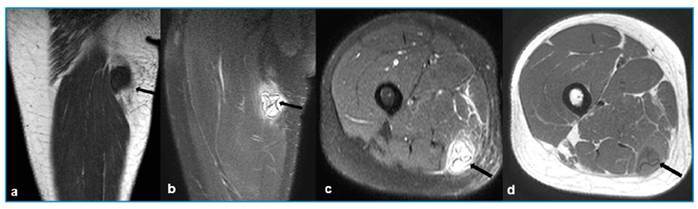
a-b, MRI of the patient’s right thigh revealed T1-weighted hypointense and T2-weighted fat-suppressed series hyperintense lesions in the semimembranosus muscle (arrow). c-d, MRI thigh shows rupture of a muscular hydatid cyst with a “water lily sign” produced by detachment of the germinal membrane of the endocyst (arrow).
